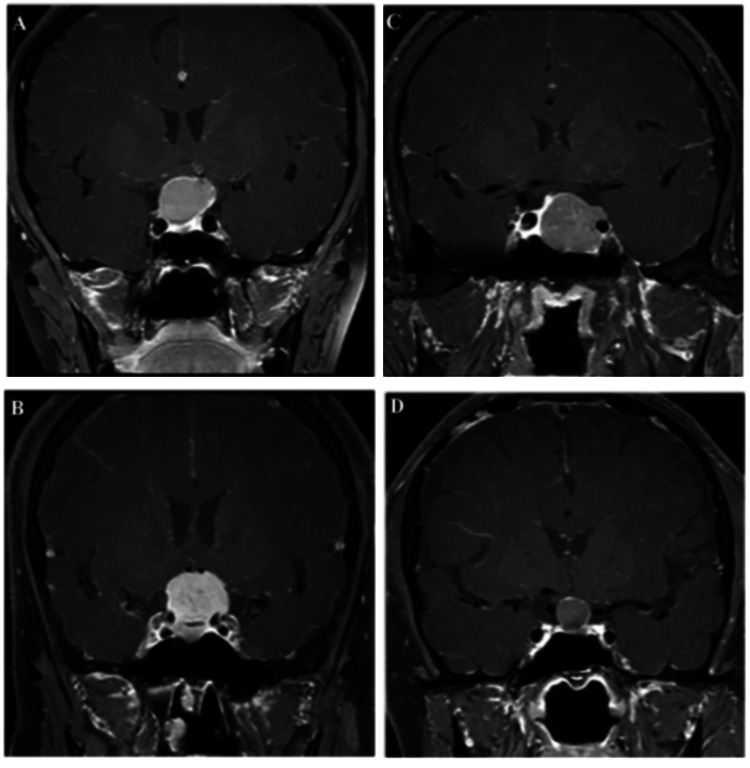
Different lesions on contrast-enhanced T1-weighted image. Permission to use was obtained from the original publishers [13]. (A) Craniopharyngioma; (B) meningioma; (C) pituitary adenoma; (D) Rathke cleft cyst.
